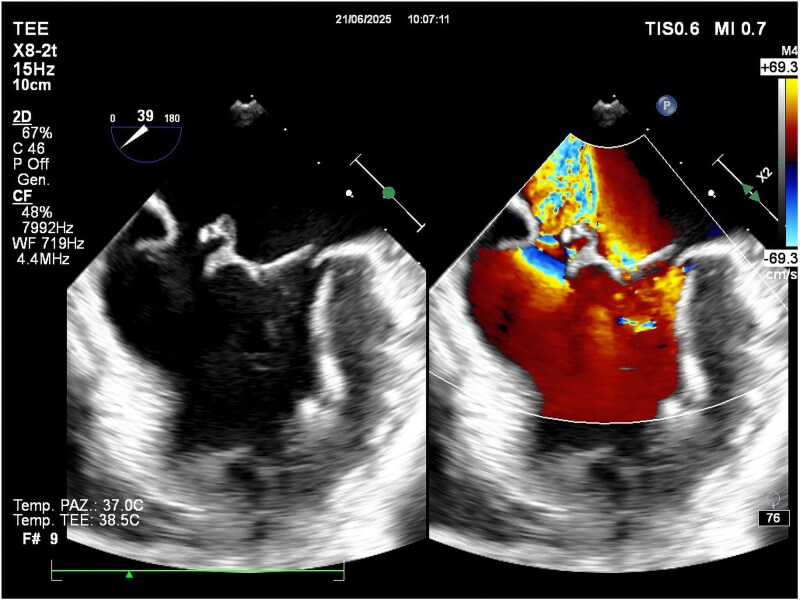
TOE bicommissural view showing flail leaflet, posterior leaflet tethering and massive mitral regurgitation at colour Doppler. TOE, transoesophageal echo.
